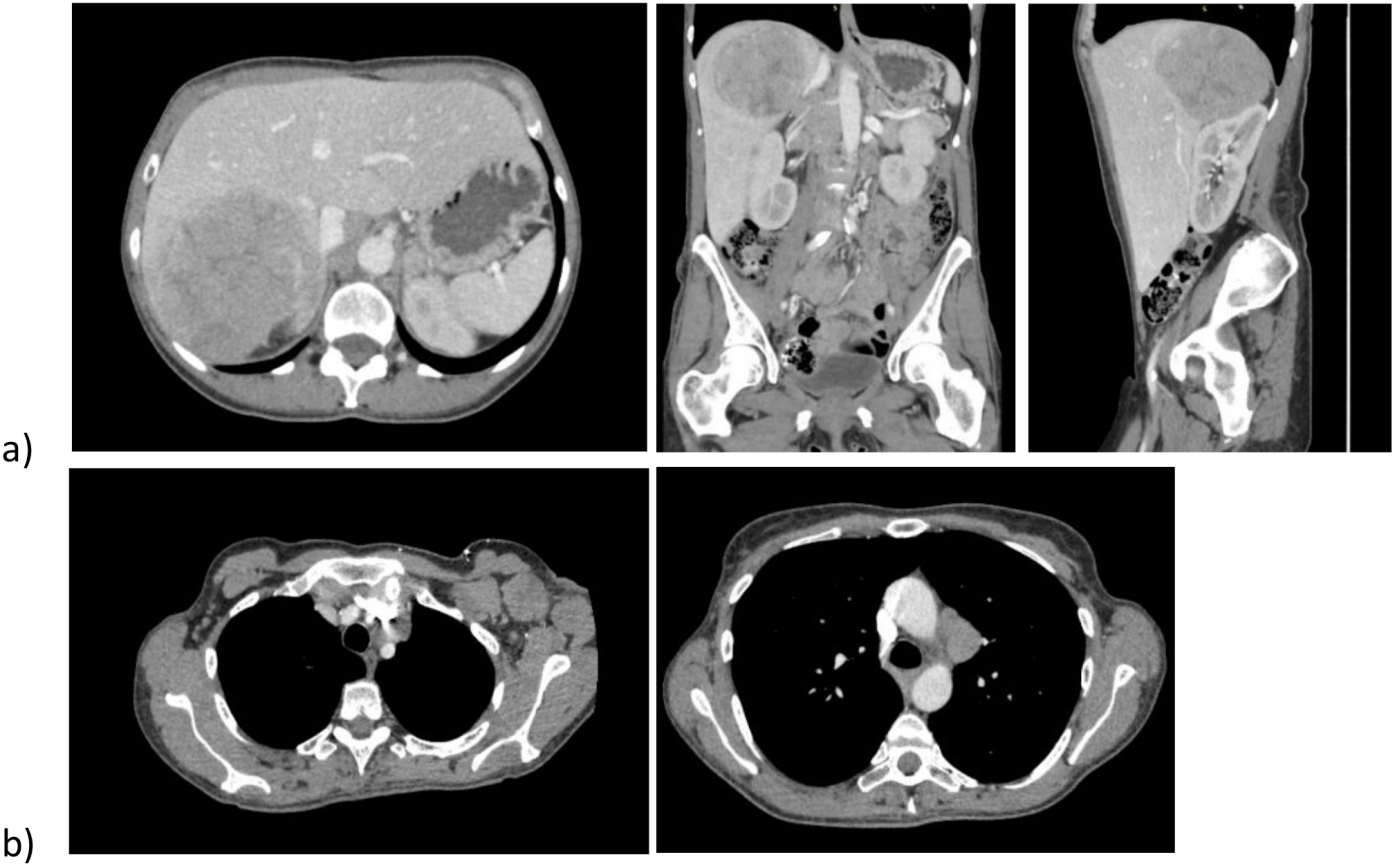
(A) CT AP with contrast 7/15/2022: new right hepatic mass up to 10 cm with new extensive retroperitoneal, periaortic, and pericaval adenopathy. (B) CT Chest 7/15/2022: new left axillary (up to 3.9 cm) and mediastinal adenopathy (up to 3.4 cm).
